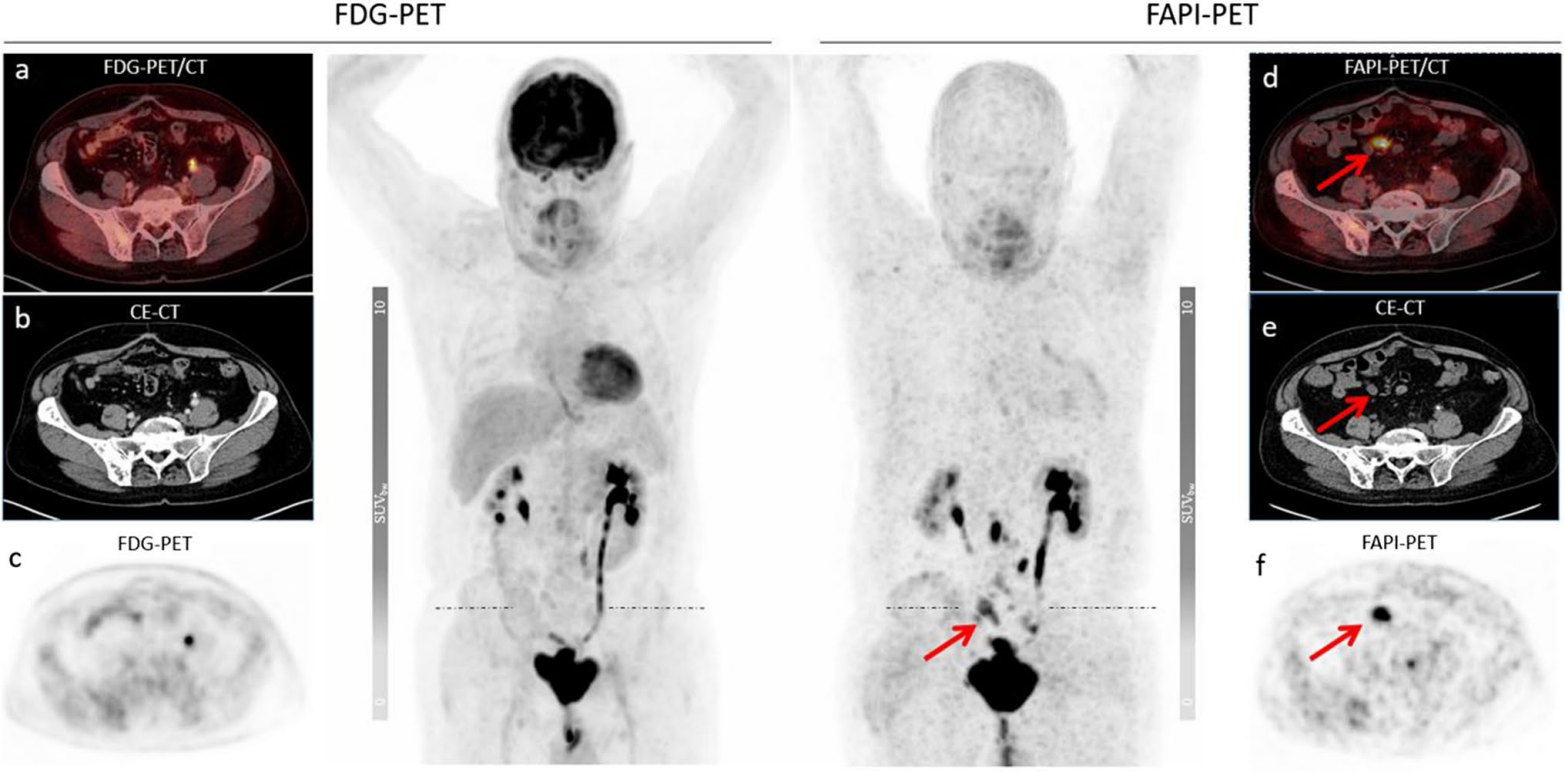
68-year-old patient in the 2^nd^ year of the follow-up after radical cystectomy with local recurrence und restaging with PET/CT scan. The PET/CT scan reveals multiple enlarged abdominal lymph node metastases, i.e., mesenteric lymph node metastases, which are only [^68^ Ga]FAPI-avid (d–f) [^68^ Ga]FAPI-SUV_max_ 10,11 vs. [^18^F]FDG-SUV_max_ 3,16) (red arrow = FAPI)
